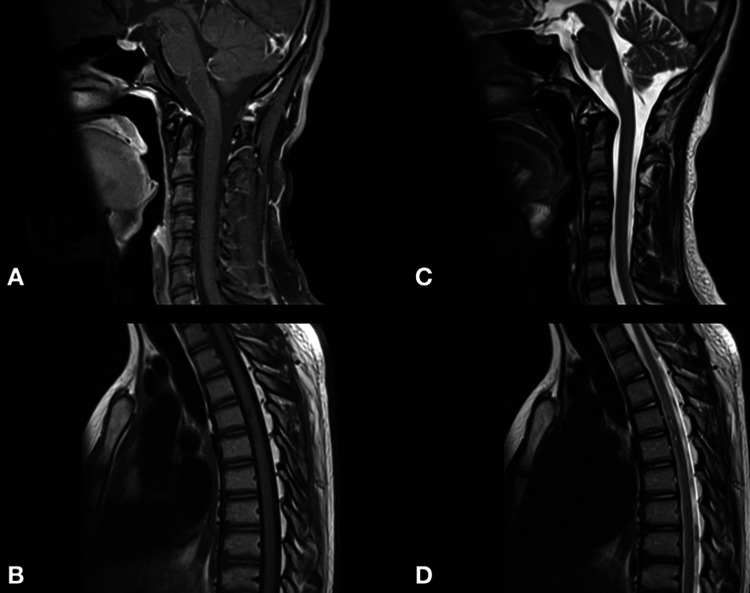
Follow-up T1-weighted (A, B) and T2-weighted (C, D) MRI scan of the cervical and thoracic spine revealed resolution of the hemorrhagic changes.
